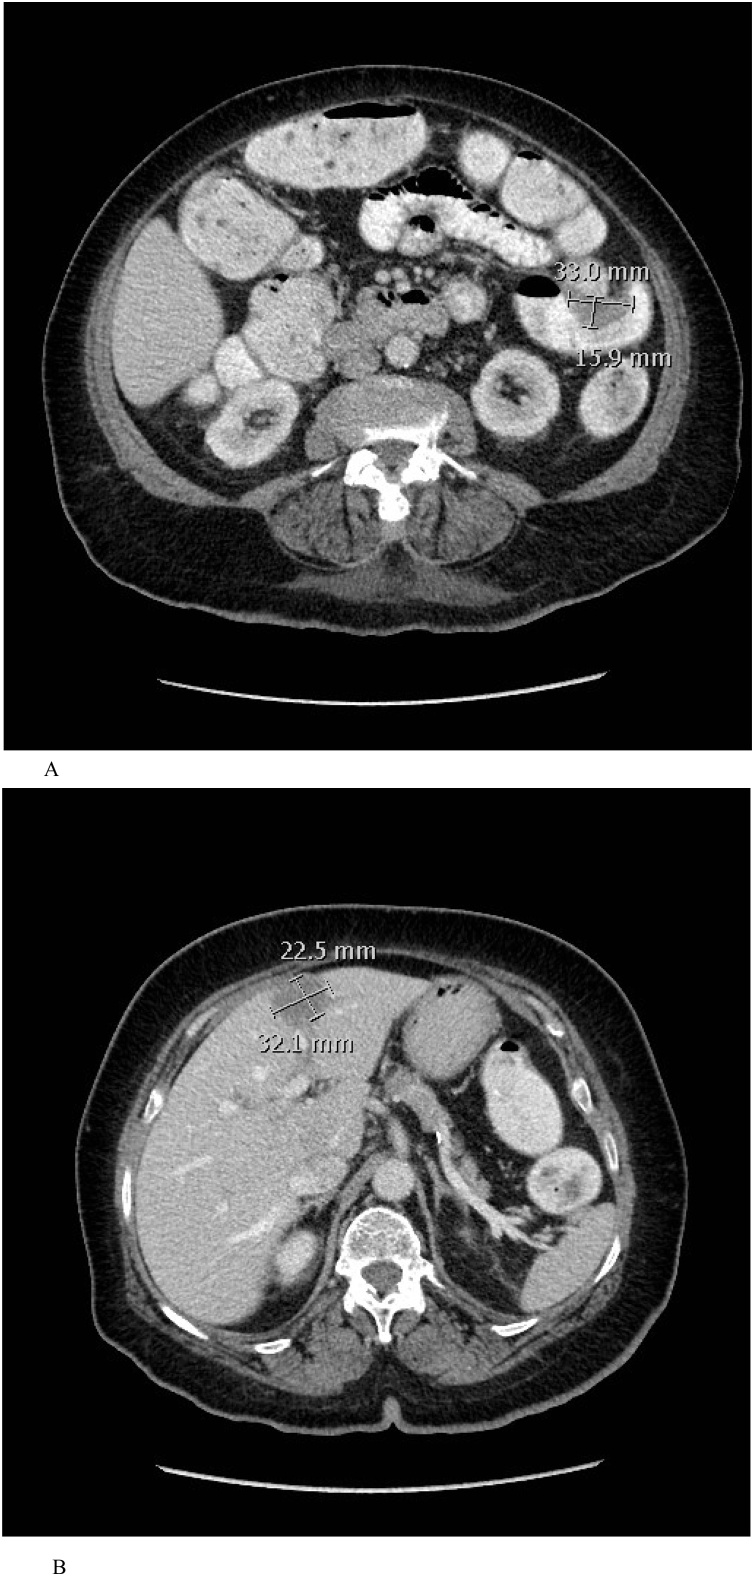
A: CT scan of the chest, abdomen, and pelvis with contrast showed a focal polypoidal thickening seen at the proximal sigmoid colon extending 3 cm long with a maximum wall thickness of 2 cm. B: CT scan of the chest, abdomen, and pelvis with contrast showed a few hypodense nodules in the liver, the largest in segment IV, measuring 3.4 × 2.7 cm, and highly suspicious for liver metastasis.
